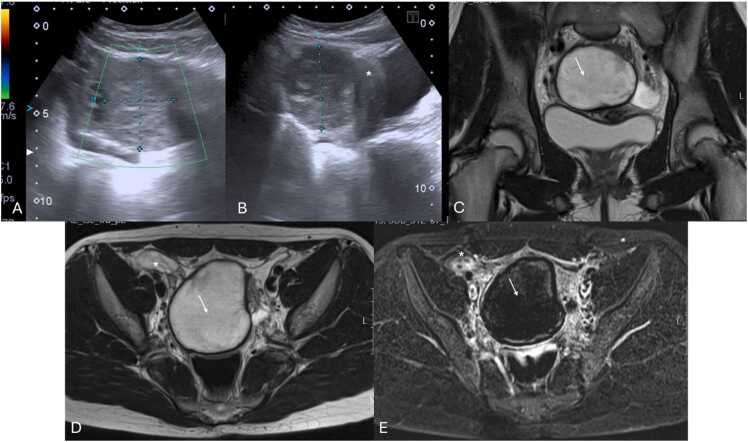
(A and B) Suprapubic Pelvic Ultrasound with Color Doppler performed in the emergency department on a 12-year-old female, revealing an enlarged left ovary (between calipers) located in an abnormal position, adjacent to the uterine fundus (*), with a heterogeneously abnormal echotexture and loss of vascular flow. Given the nonspecific imaging findings, MRI imaging was obtained. Coronal and axial T2-weighted (C and D, respectively) as well as T1-weighted Fat Suppressed (FS) post-contrast subtraction sequence (E) images confirming the presence of an enlarged left ovary (arrows), abnormally positioned in the midline, with loss of follicles and lacking parenchymal enhancement. In (D and E) please note the right ovary with preserved volume, parenchymal follicles and normal enhancement.
